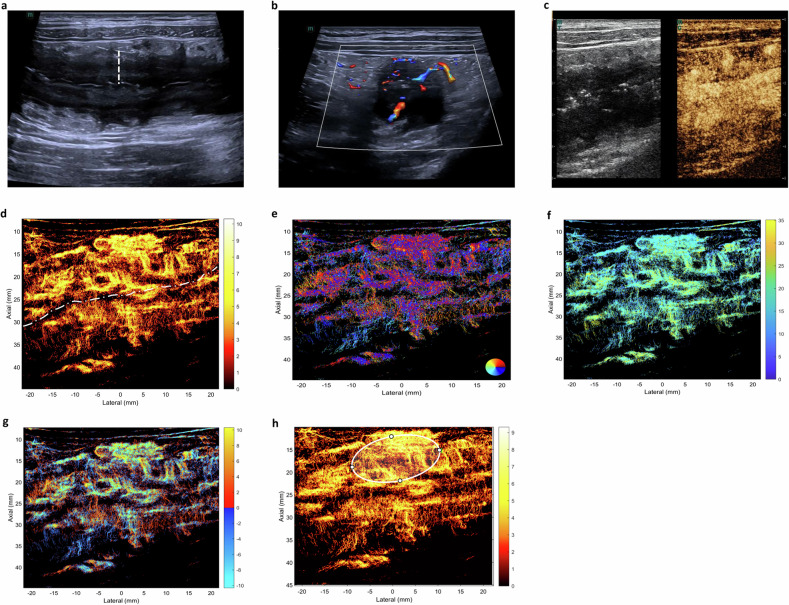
A 24-year-old female patient diagnosed with active Crohn’s disease (CD). The value of total simple endoscopy score for CD (SES-CD) is 13. On longitudinal scan, the bowel wall layers are disrupted. The bowel wall thickness of the descending colon is 11.0 mm (white dashed line, a); color Doppler signal shows longer linear blood flow signals extending to and connecting with the mesentery within the inflamed bowel wall (Limberg IV, b); contrast-enhanced ultrasound (CEUS) of the inflamed bowel wall displays complete enhancement of the bowel wall from the mucousal to serosal layer (pattern I, c); super-resolution CEUS (SR-CEUS) vascular density map of the inflamed bowel wall shows disorganized, tortuous vascular morphology, enlarged vessels and fine branching vessels the inflamed bowel wall. The white dashed line demarcates the intestinal lumen (d). The SR-CEUS vascular density direction map of the inflamed bowel wall is shown. Brighter colors indicate higher vascular density (e). The SR-CEUS flow velocity map of the inflamed bowel wall is shown. Variations in color on the scale bar represent different blood flow velocities (f). The SR-CEUS flow direction map of the inflamed bowel wall is shown. On the color scale, positive values are assigned to flow toward the probe, and negative values represent flow away from it (g). By SR-CEUS offline analyzing, quantitative parameters were automatically derived from a manually placed region of interest (ROI) over the inflamed bowel wall for objective assessment (h)
